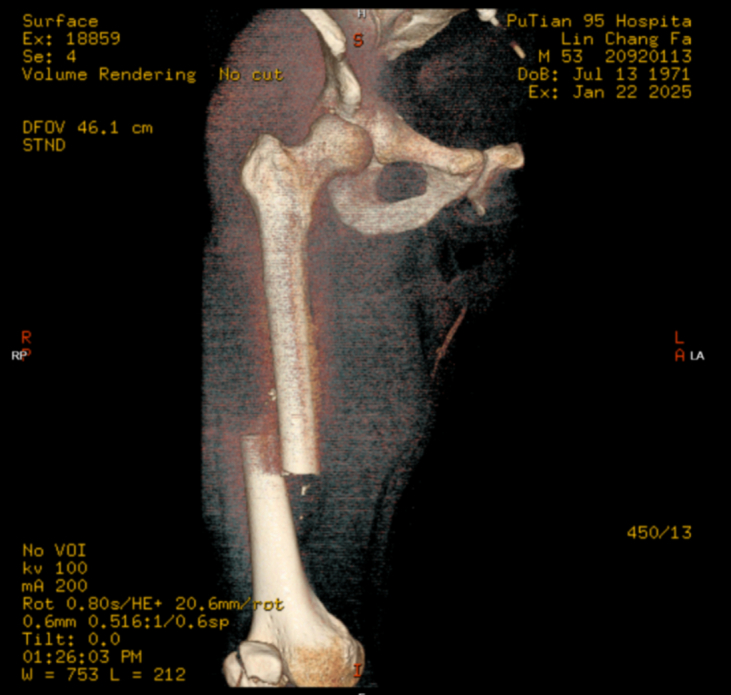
Preoperative CT identified a distal femoral shaft fracture (OTA/AO 32A) and a patellar fracture (OTA/AO 34-C3).
